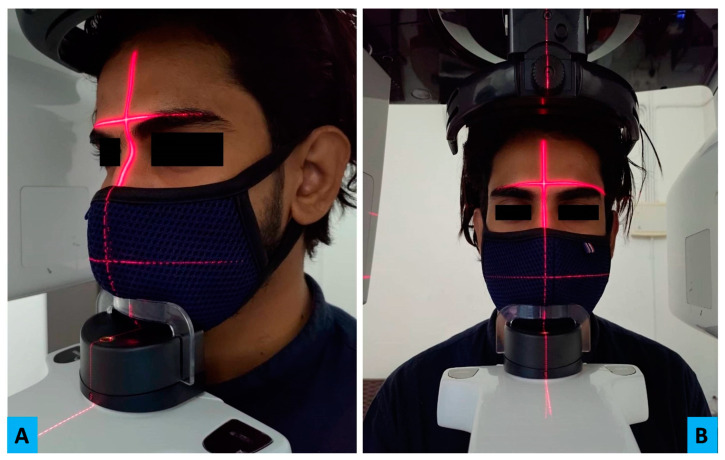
This figure depicts a patient’s lateral (A) and front (B) profile while being prepared for cone beam computed tomography (CBCT) imaging. Here, patient is shown wearing the face mask as a part of “new” infection control protocol. The red colour positioning lights can be seen assisting in the positioning of patient in the correct Sagittal and coronal plane.
